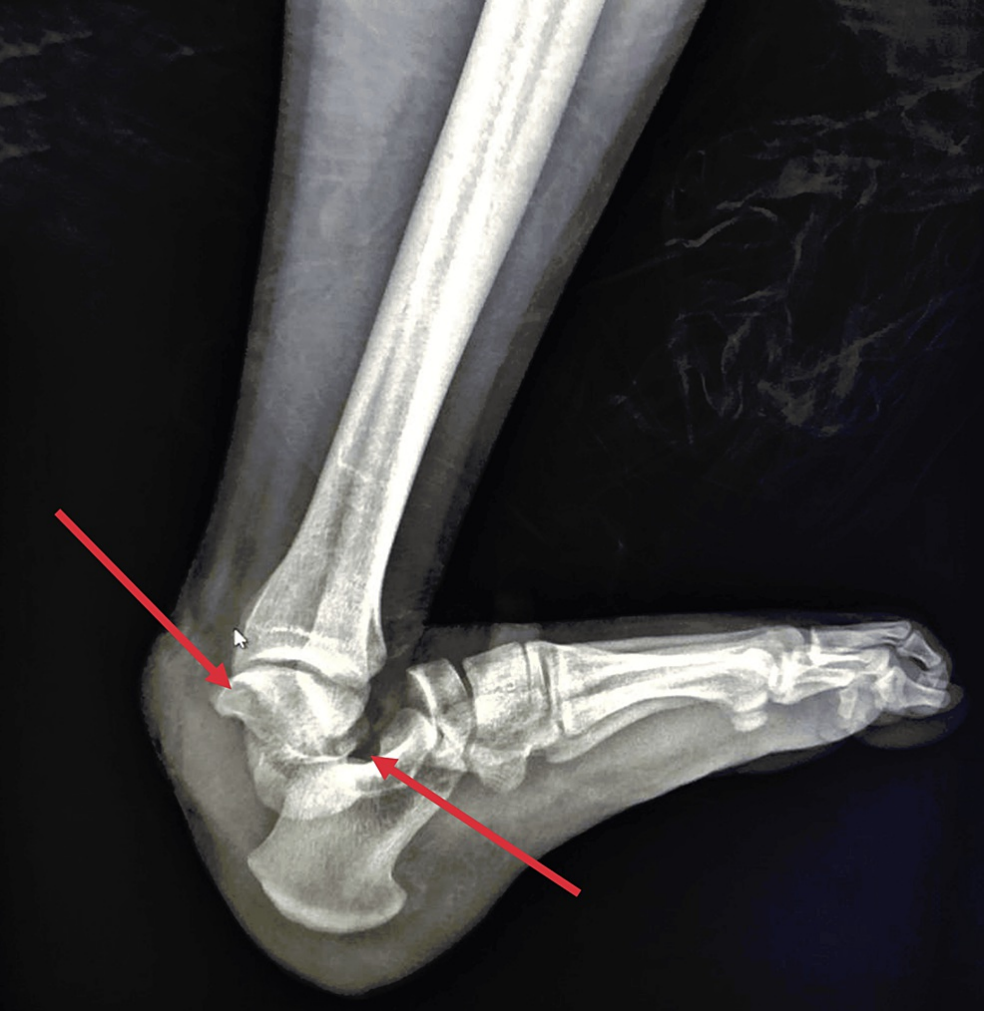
Lateral radiographic view of the left ankle. The arrows designate the subtalar dislocation and the medial rotation of the talus.
